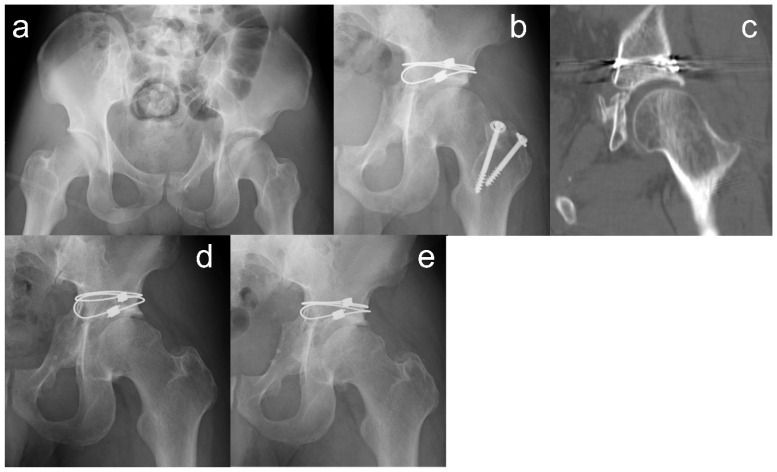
A case presentation of a 28-year-old man injured by a fall from a height. (a) Preoperative plain radiograph showed a dual-column acetabular fracture. (b,c) Plain radiograph and computed tomography at 6 months after surgery. The articular reduction quality is satisfactory and that of the anterior column is poor. (d) Plain radiograph at the ten-year follow-up. No osteoarthritis or heterotopic ossification were seen. (e) Plain radiograph at the last follow-up. The hip pain appeared approximately 12 years after surgery, and Tönnis grade I osteoarthritis was observed.
